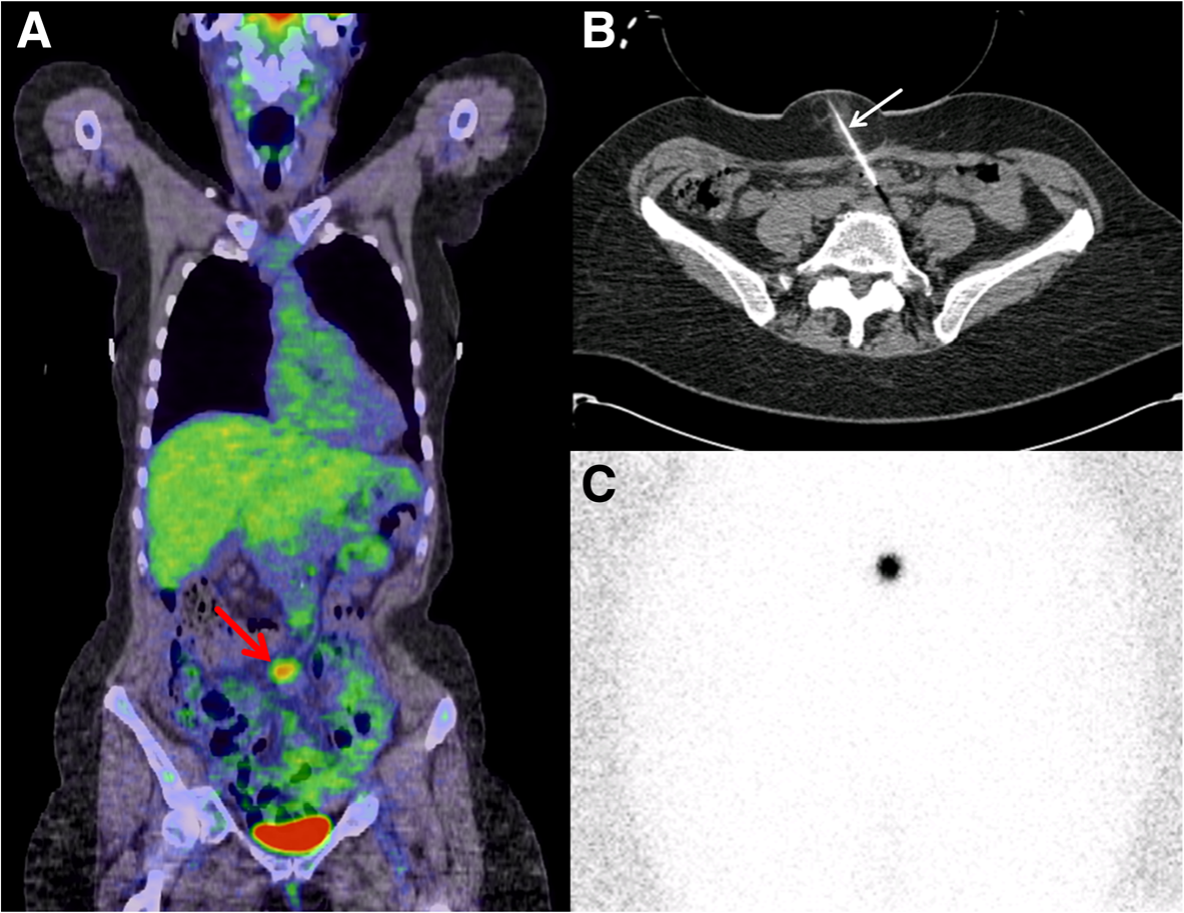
A 72-year-old female with high-grade serous ovarian carcinoma. Six years after surgical resection and chemotherapy, rising serum CA-125 prompted a F-18 fluorodeoxyglucose positron emission tomography/computed tomography (FDG PET/CT) exam. Coronal fusion FDG PET/CT (a) depicts a hypermetabolic lymph node in her mesentery (red arrow) that was suspicious as a solitary site for metastatic disease. Given concern for difficulty palpating this node intraoperatively, radiolocalization was performed. Using CT guidance (b), a compression device with hollow center displaces overlying bowel as the Tc-99m MAA injection needle (white arrow) is advanced to the target lymph node for perilesional injection. Excellent localization was confirmed by anterior pelvic scintigram (c). This lymph node was surgically removed and confirmed metastatic disease, prompting initiation of chemotherapy
